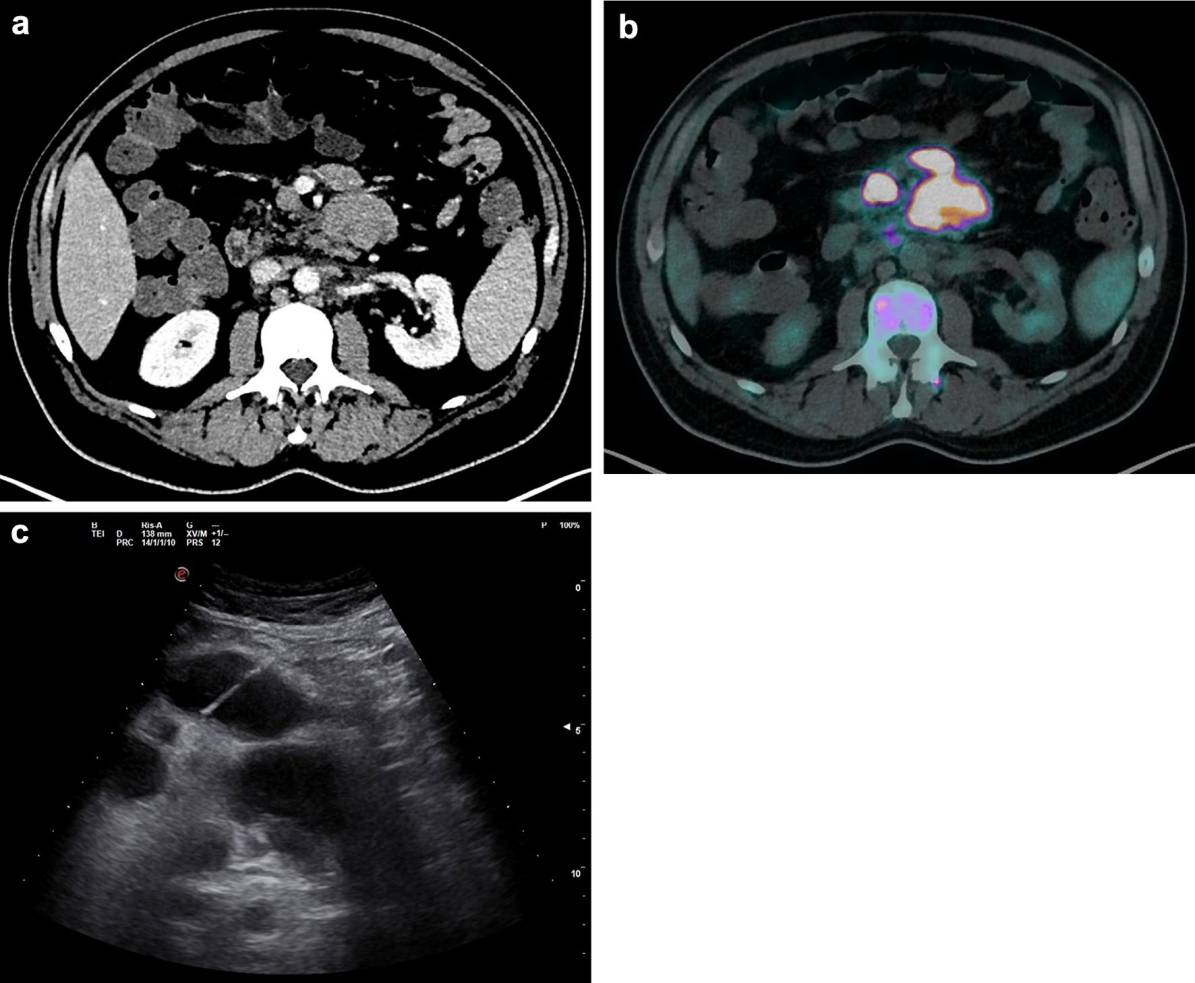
Core needle biopsy diagnosis of large B cell lymphoma in a patient with large mesenteric lymph nodes. Preoperative contrast-enhanced CT (A) and [18F]FDG PET/CT (B) images were evaluated to define the most favorable target for percutaneous biopsy. A transabdominal ultrasound-guided percutaneous core needle biopsy (C) was performed with a 18G semiautomatic guillotine needle and provided the definitive diagnosis
